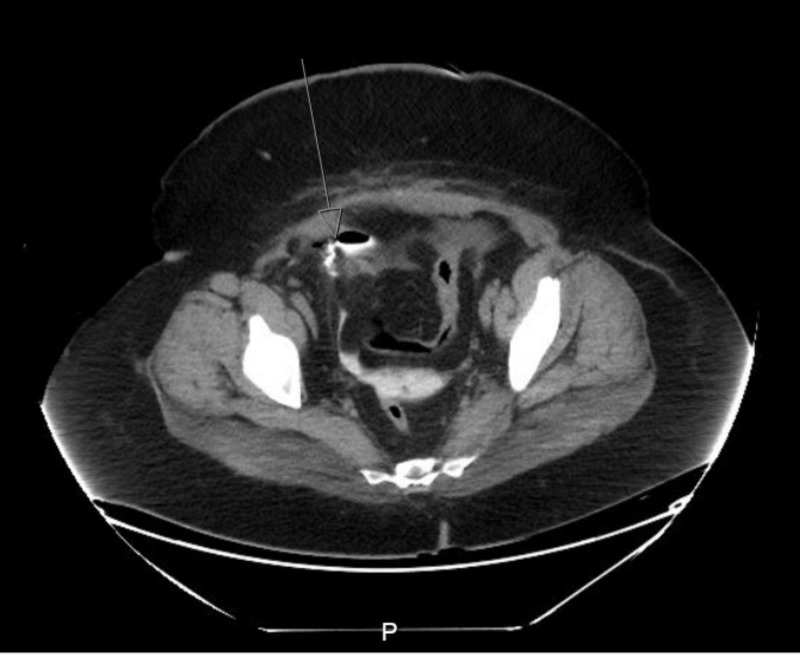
CT cystogram showing extravasated contrast within the pelvis (arrow)
